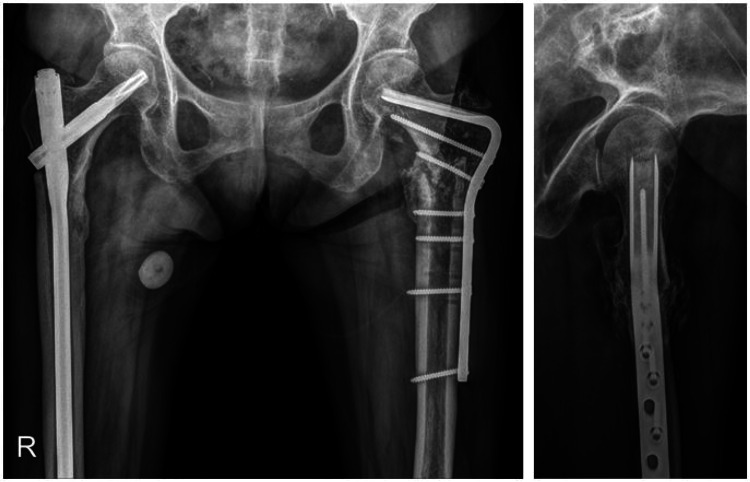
Simple radiographs seven months after re-revision surgery shows connection of fracture with external callus.
